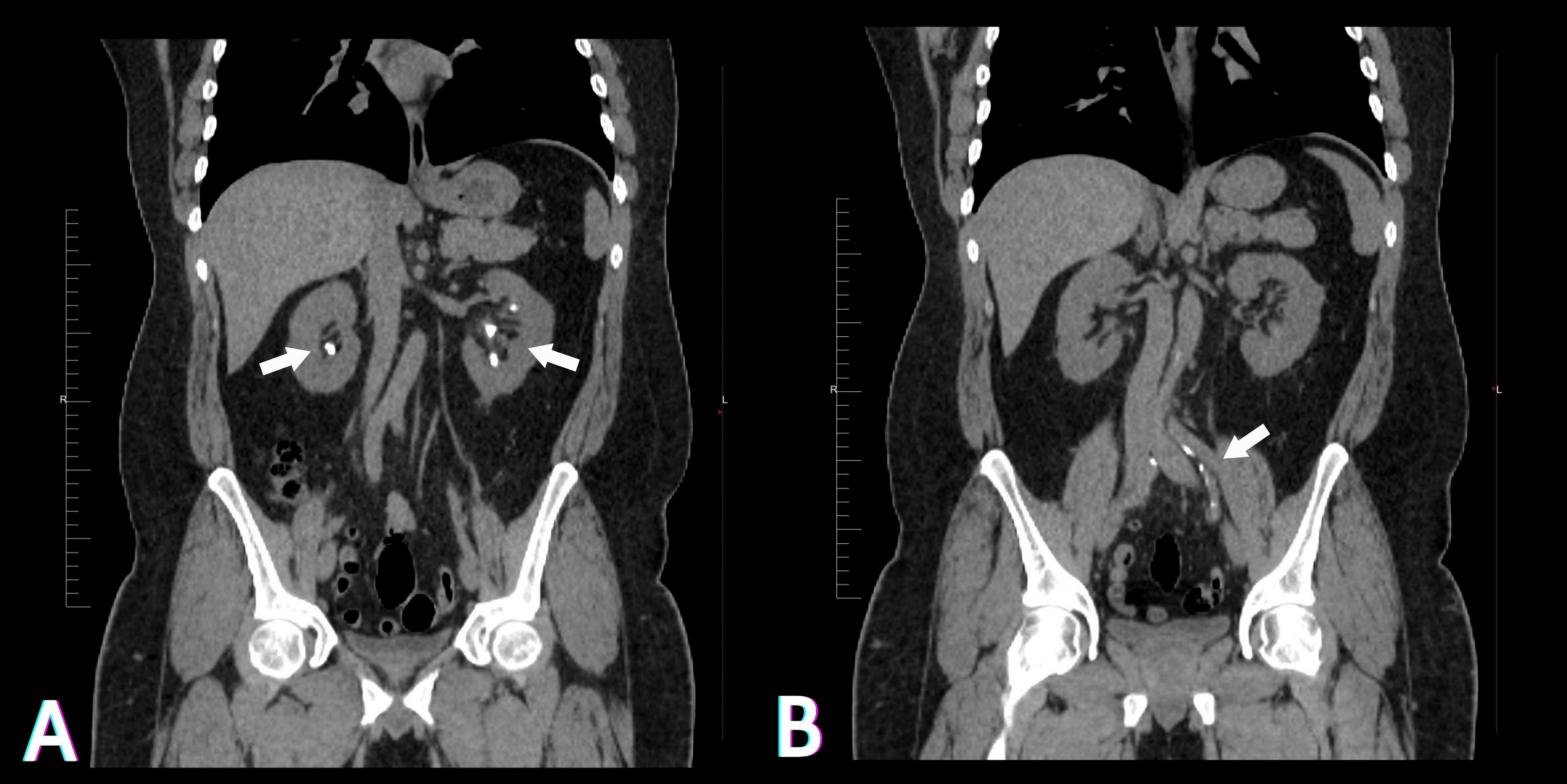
Coronal non-enhanced CT scan of the abdomen and pelvis. (A) Multiple bilateral non-obstructive renal calculi; (B) calcifications in the left common iliac artery.
